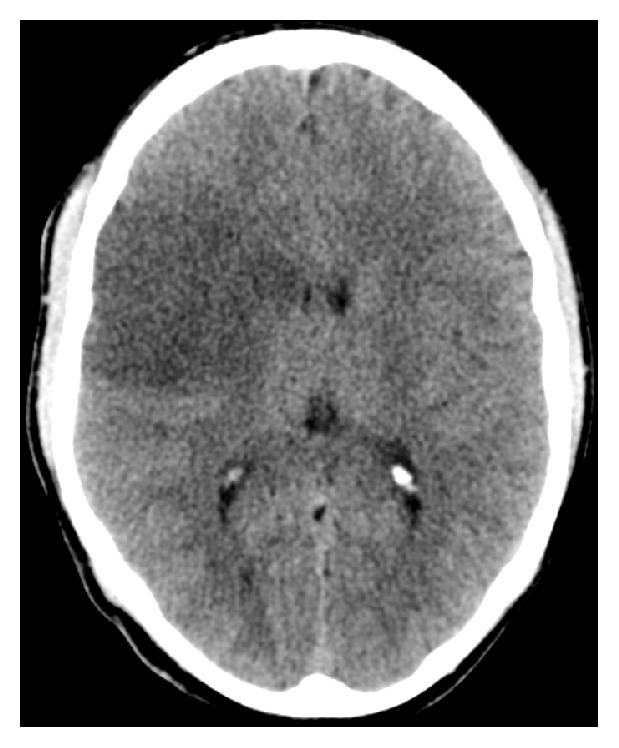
Computed tomography of the brain showing a cerebral infarction in the right hemisphere.
